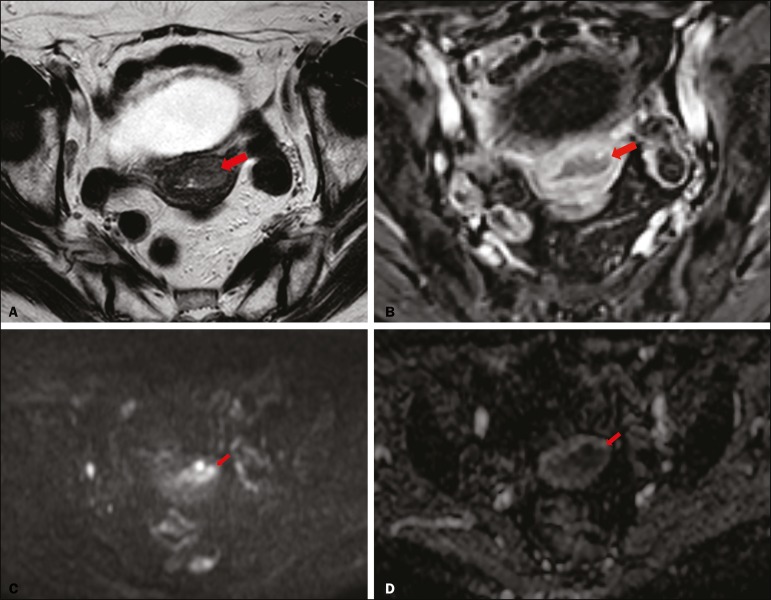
MRI of a 78-year-old woman with endometrial cancer. A: Axial
